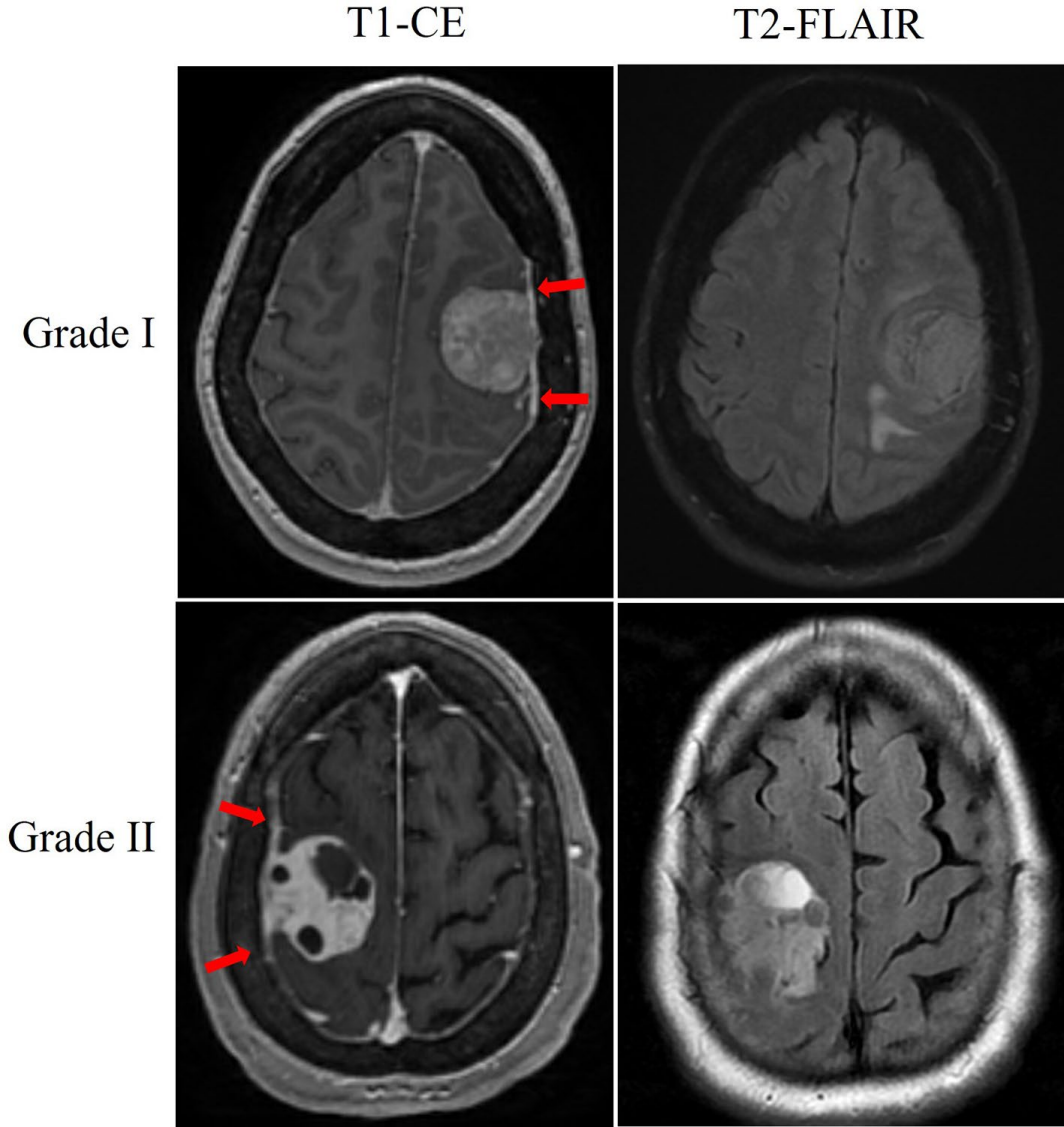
Meningioma with typical radiological signs for Grade I and Grade II. T1-CE MRI after gadolinium injection depicts heterogeneous contrast enhancement. The red arrows show the dural tail on T1-CE.
